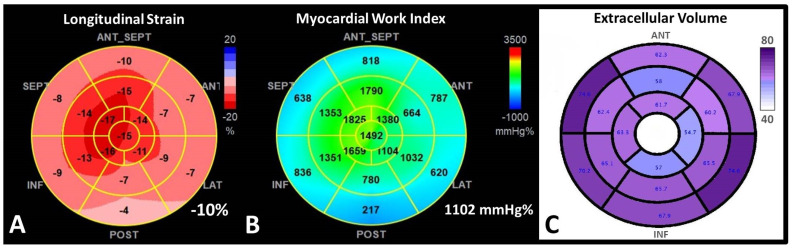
Apical sparing pattern in ATTR cardiac amyloidosis. (A) Longitudinal deformation bull’s eye plot of the left ventricle by speckle−tracking−echocardiography in a 46-year-old patient with ATTR-CM with significantly decreased global LS of −10%, with longitudinal deformation impaired at the basal, midventricular segments, and relatively preserved at the apex, in an “apical sparing” pattern. (B) The corresponding myocardial work index (MWI) bull’s eye plot, confirming a similar apical sparing pattern, with preserved MWI in the apical segments; (C) Extracellular volume (ECV) bull’s eye plot evaluated by cardiac magnetic resonance, using a 16-segment model, displaying a progressive decrease in the ECV from base to apex, explaining the substrate of apical sparing deformation pattern.
